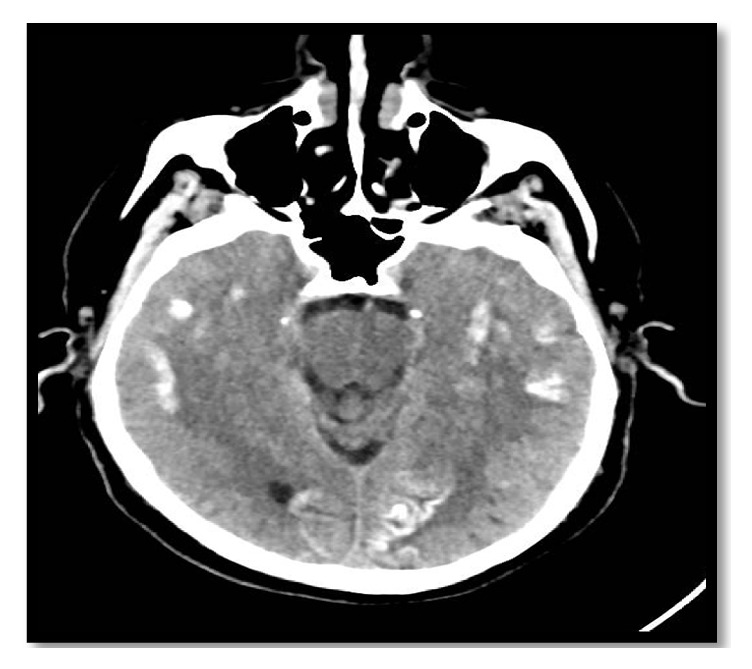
Postictal plain CT of the brain showing no interval abnormality compared to the CT of the brain upon presentation. Abbreviation: CT, computed tomography.
